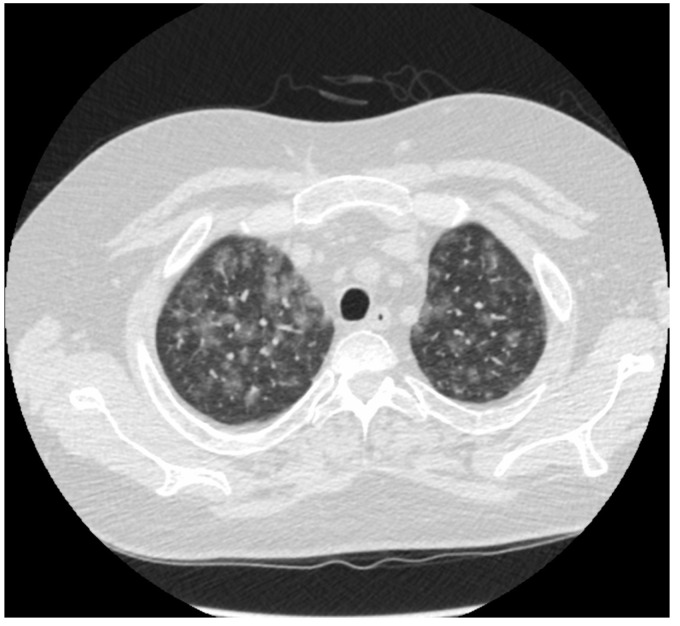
Chest HRCT, axial planes, showing numerous bronchiolocentric GGO, with symmetric distribution.
